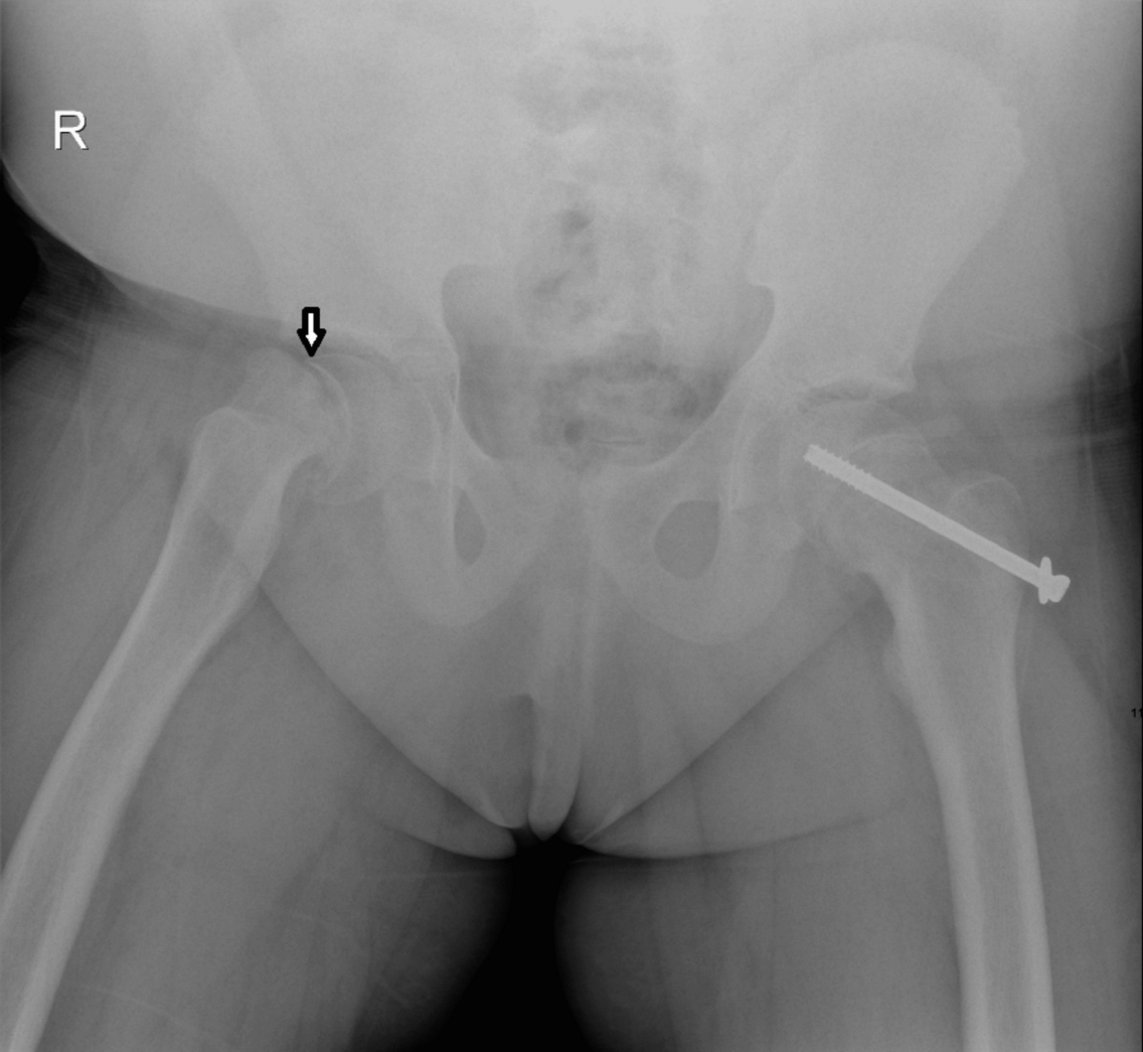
X-ray pelvis (AP view) showing SUFE (white arrow) in the right hip and previous percutaneous pin fixation in the left hip. SUFE: Slipped Upper Femoral Epiphysis; AP: Anteroposterior
